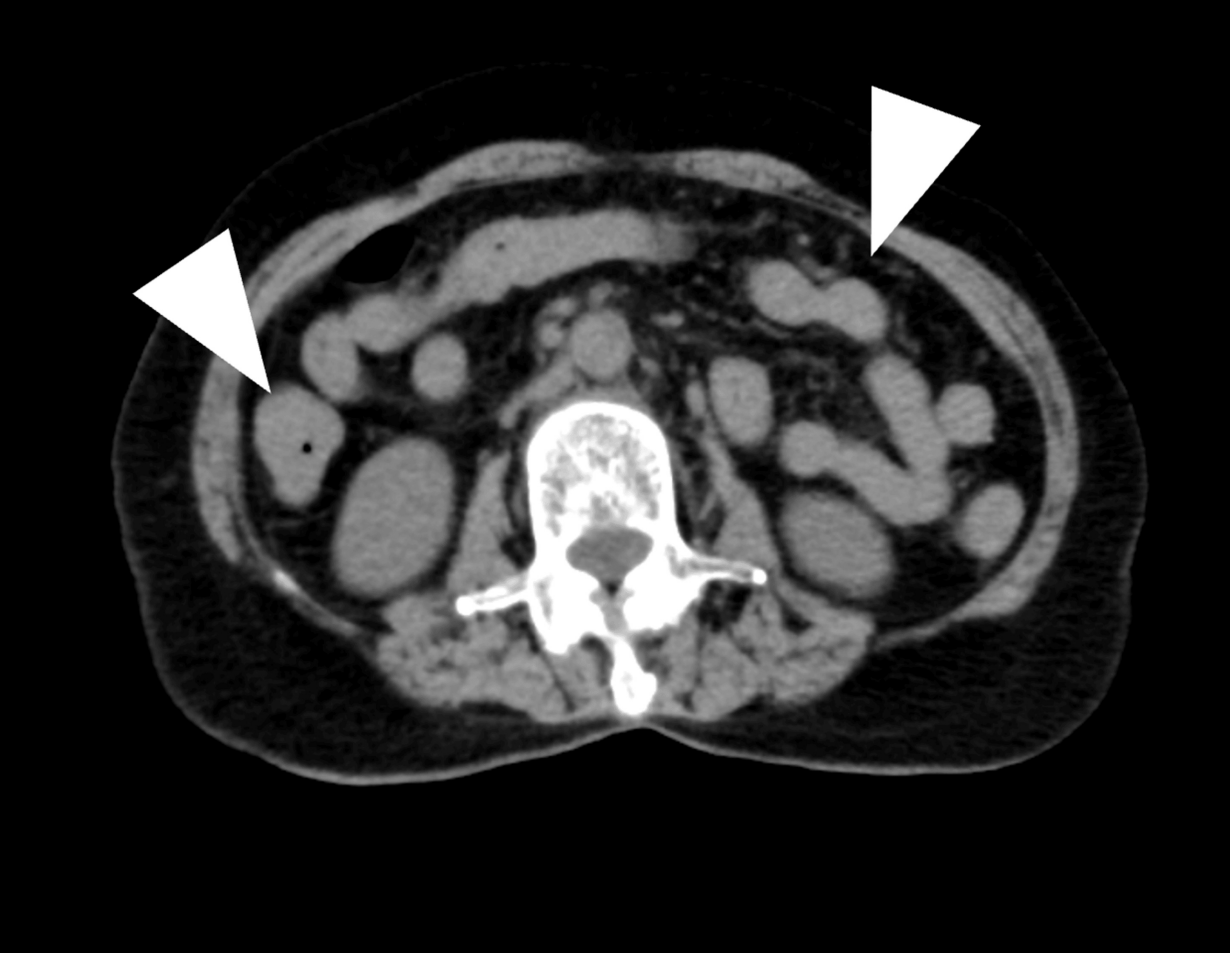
Abdominal CT in the first episode Abdominal CT performed during the first episode demonstrated subtle bowel wall edema, indicated by the white arrow, suggesting gastrointestinal involvement. CT: computed tomography
